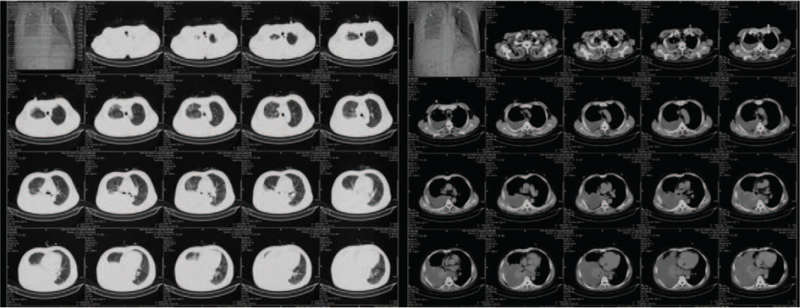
A large, dense mass involving the middle and inferior lobes of right lung can be observed, along with pleural thickening and pleural effusion in the nonenhanced CT imaging of chest. CT = computed tomography.
